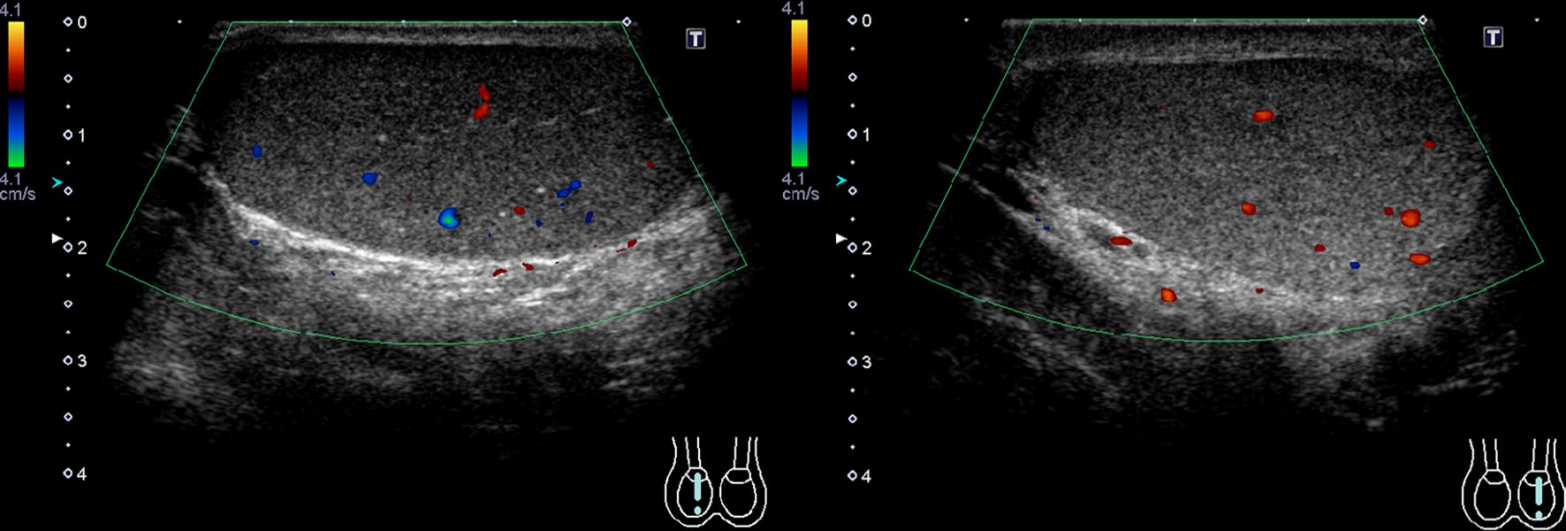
Ultrasound findings after 6 months from the operation. Six months after operation, surface ultrasound scan of the scrotum showed sufficient testicular perfusion and volume. There was no evidence of atrophy
